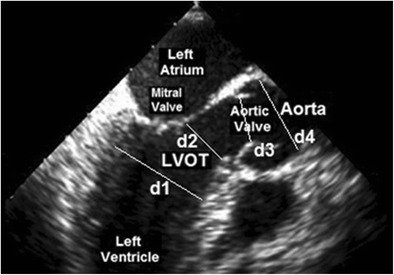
Apical 2 chamber view of the left ventricle demonstrating the LVOT, the aortic valve and the aorta, and the different diameters at each point d1 to d4
